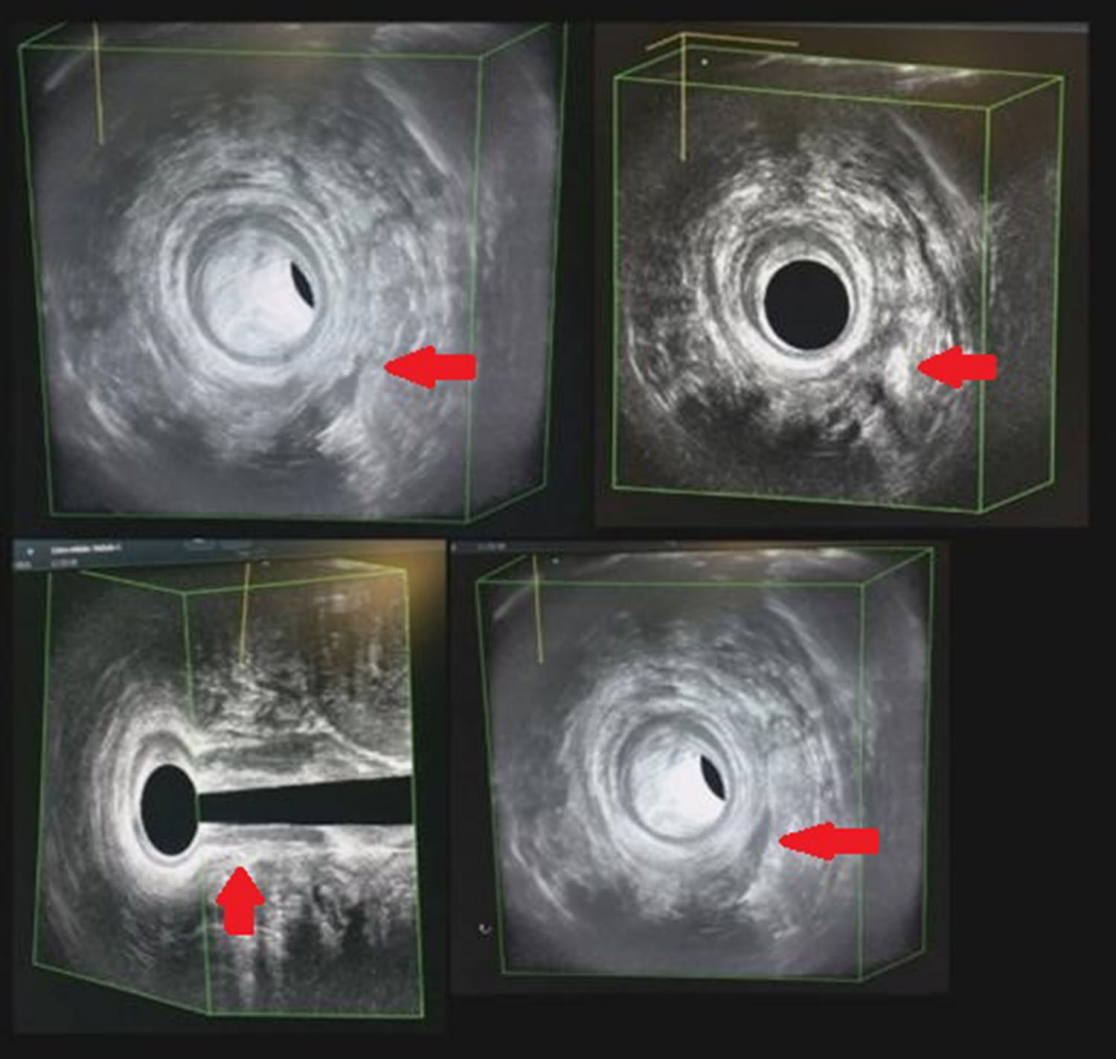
Transanal ultrasound follow-up showing complete healing of the fistula (red arrow) at 6 months after surgery
